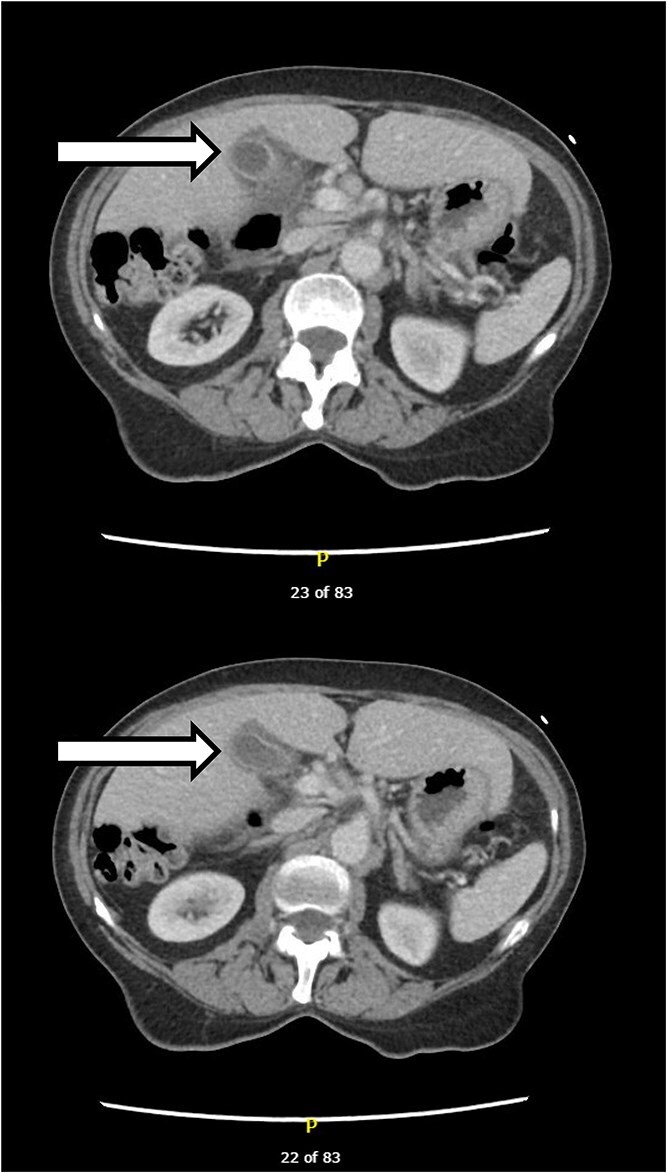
CT findings concerning for acute cholecystitis at the patient’s initial presentation.
